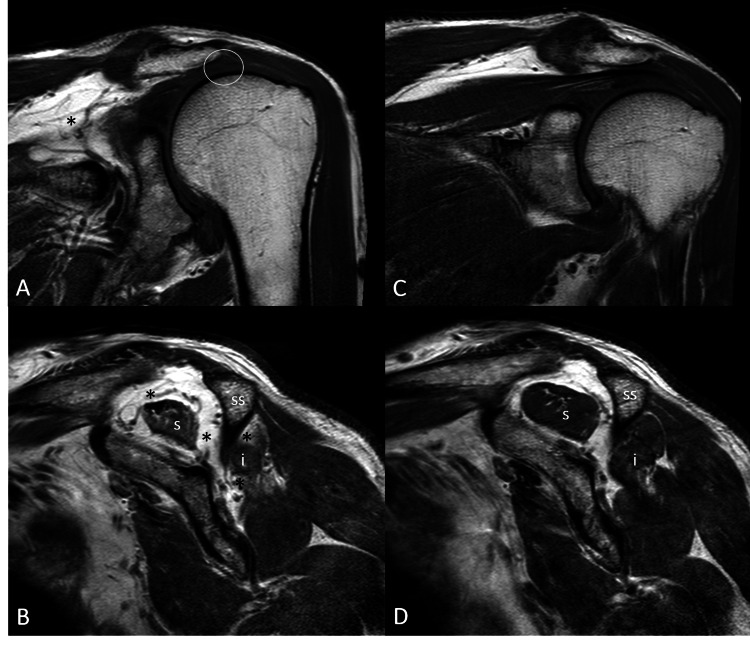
Absence of supraspinatus tendon (circle) on a coronal (A) and sagittal (C) T1-weighted image. This is due to the massive rotator cuff tear involving nearly the entirety of the supraspinatus (s) and infraspinatus (i) tendons. Both muscle bellies demonstrate progressive severe fatty atrophy (*) which was not present in a previous MRI a year earlier (C and D). ss: scapular spine
